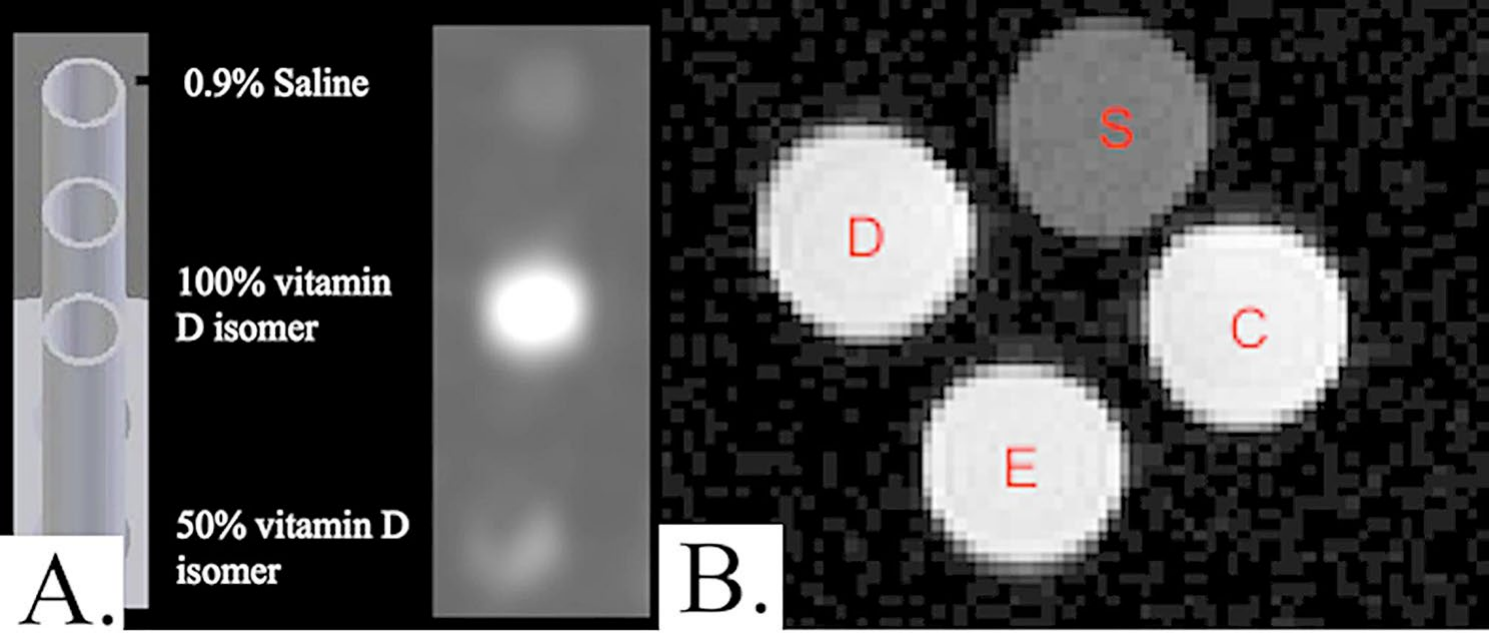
(A) MR acquisition showing saline, undiluted, and diluted vitamin D relative to positioning on the 3D printed cylinder and (B) Visual comparison MR images of saline (S) to the three vitamin D isomers 100% solutions- D: Cholecalciferol; E: Ergocalciferol; C: Calcitriol
